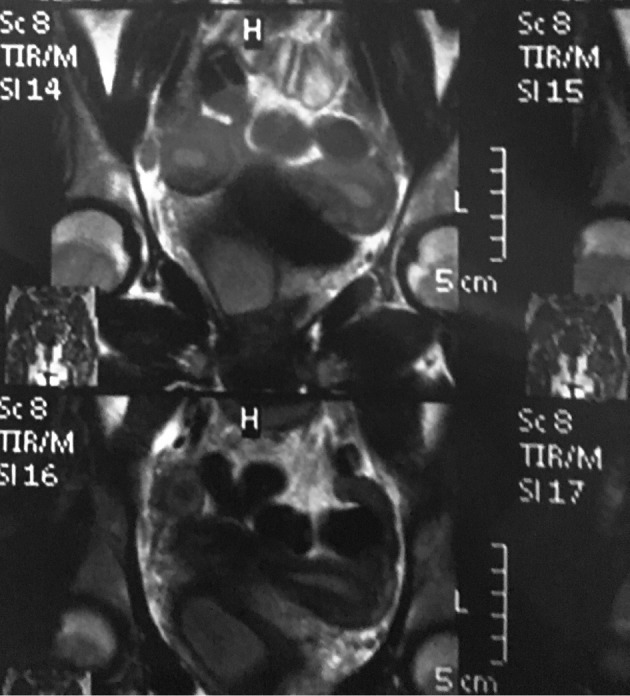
MRI image showing the bicornuate uterus and the hematocolpos
